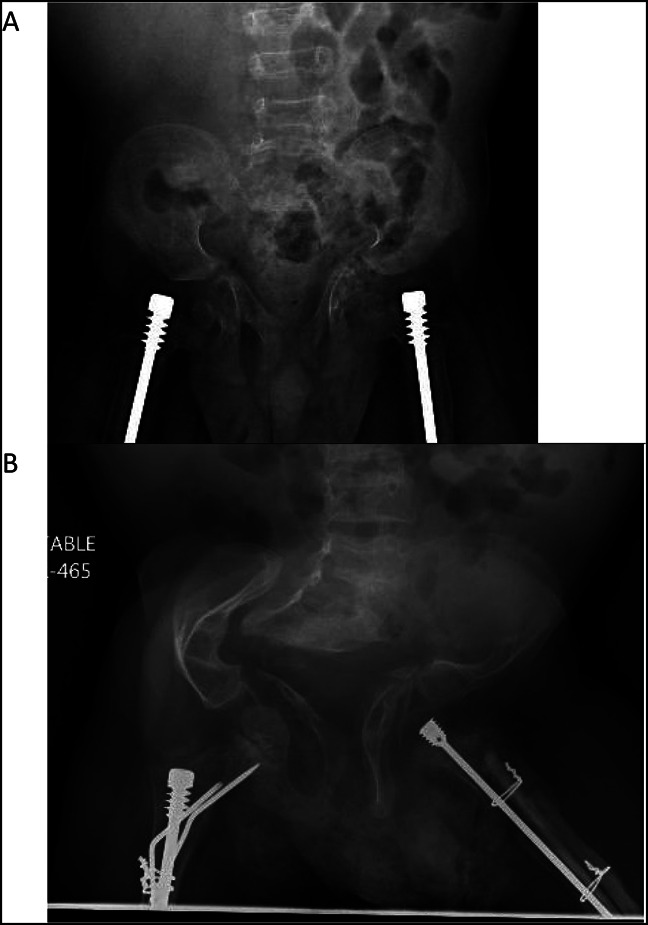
Radiograph of A, AP pelvis at 2 years and (B) AP pelvis at 8 years demonstrating marked progression of the patient's pelvic deformation and acetabular protrusio.
